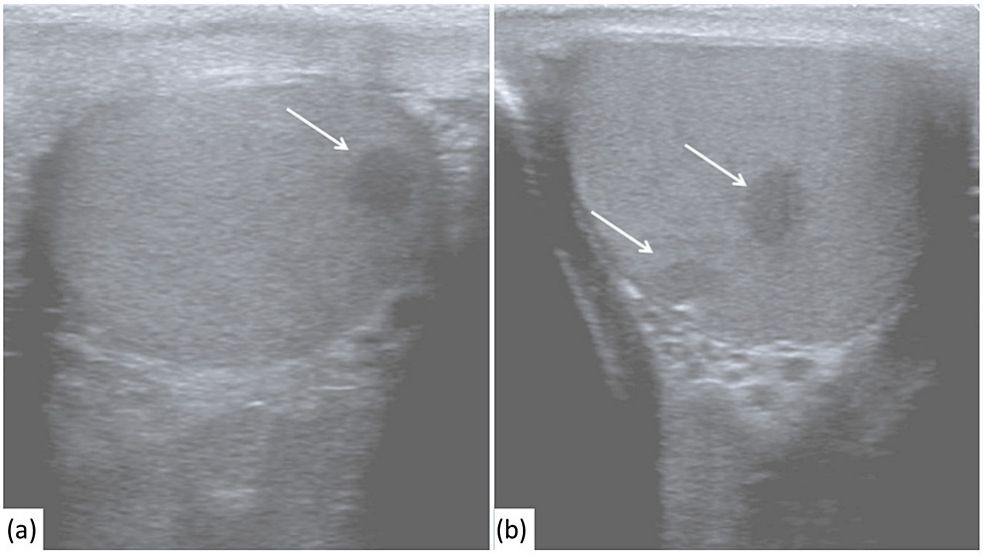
Scrotal ultrasonography with multiple rounded hypoechoic lesions in both testes (a, left testis; b, right testis).
